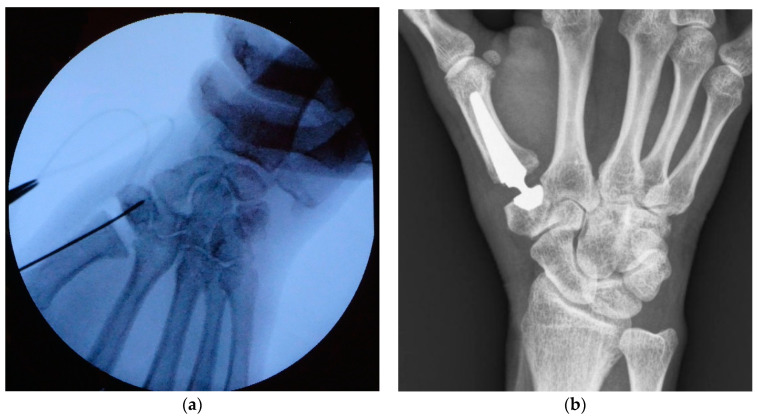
Intraoperative fluoroscopy showing Kirschner wire positioning before reaming (a); X-ray of TOUCH^®^ prosthesis taken at 1 year follow-up (b).
